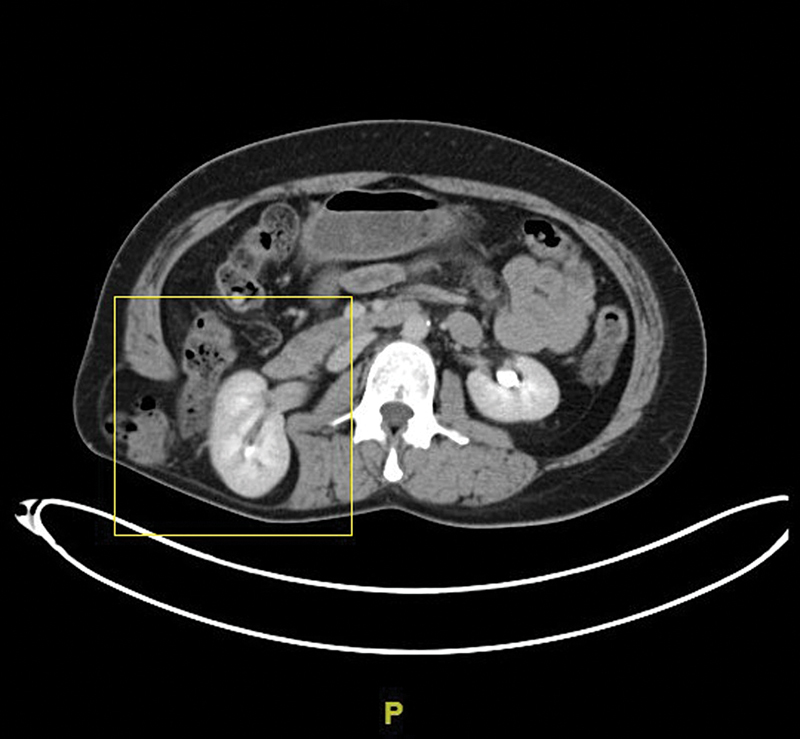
CT scan of abdomen demonstrating right lumbar hernia with colon and kidney as contents.
